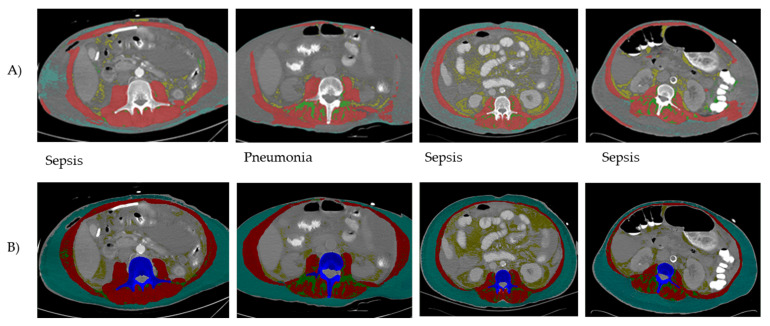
Examples of CT images following AutoMATiCA (test method) analysis (A) compared to semi-automated SliceOmatic (reference method) analysis (B). Discrepancies in tagging (i.e., coloring of different tissues) by the test method were noticed for all the abdominal tissues at this landmark (skeletal muscle and adipose tissues). The inconsistent performance of the test method was noted mainly in patients with a critical illness diagnosis.
